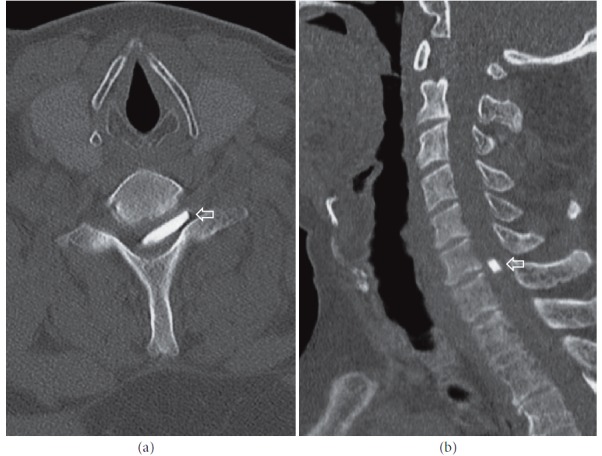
CT of the cervical spine: bone windows, axial (a) and sagittal reconstruction (b) showing a hyperdense oblong lesion at the level of C6-C7 in the left intervertebral foramen and spinal canal (arrows).
